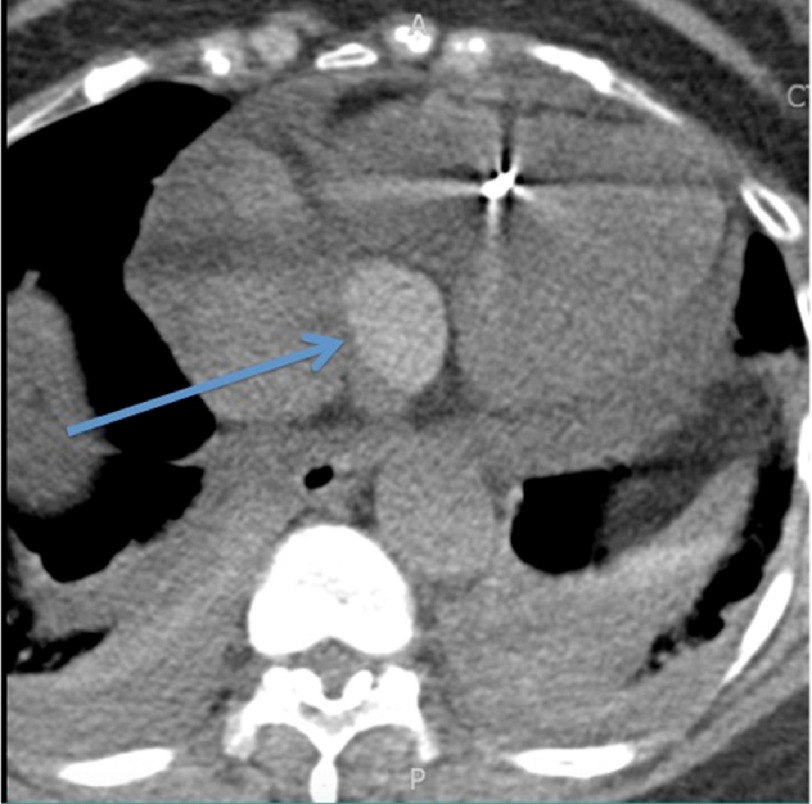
Magnified view of the hyperdense, ovoid, circumscribed structure along the inferior surface of the heart, as indicated by the arrow in this noncontrast axial slice of the CT angiogram (CTA).
